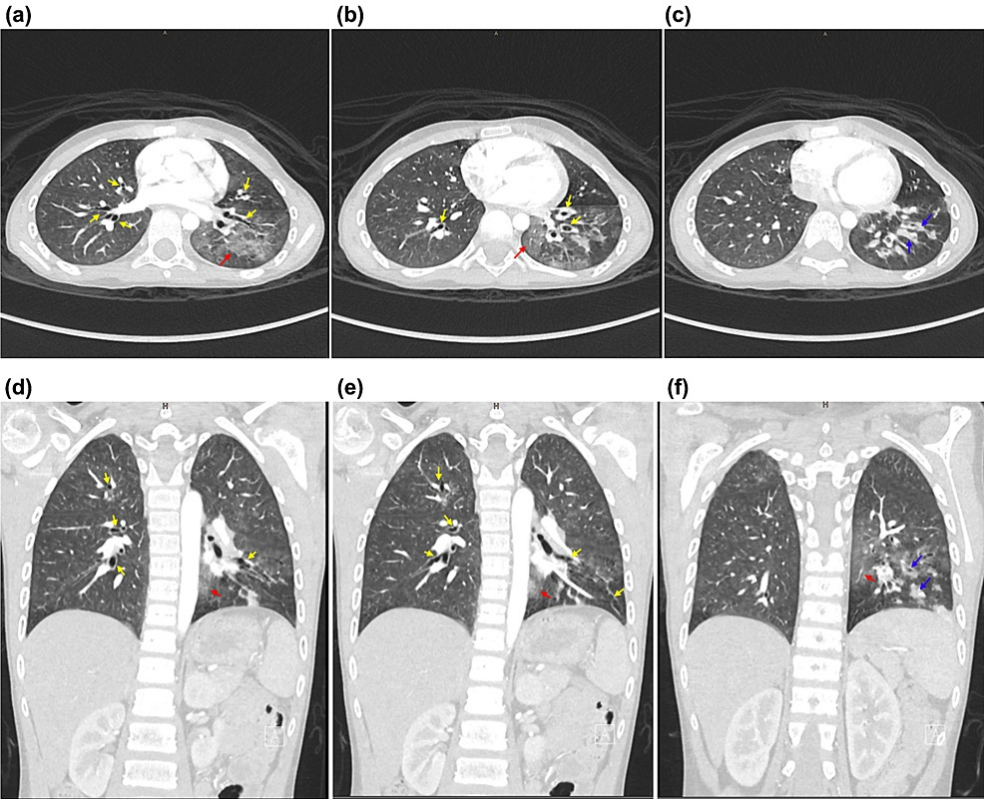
Computed tomography angiography Computed tomography (CT) showed bilateral ground-glass air-space opacity on the left side and an area of enhancement over the left lower lobe (red arrows). Bilateral cylindrical bronchiectasis affected all the lobes (yellow arrows). However, this was more prominent in the left lower lobe. Thickening of the bronchial wall was observed over the left lower lobe, and some contained fluid components (blue arrows). Three bronchial arteries were observed: one branch on the right side and two left bronchial arteries measuring 0.2 cm. Tortuosity or extravasation was not observed. Bilateral dilatation of the pulmonary arteries and bilateral hilar lymph node enlargement were observed. The largest lymph node was on the left and measured 1.5 cm. The cardiomediastinal structure and major vessels were unremarkable.
